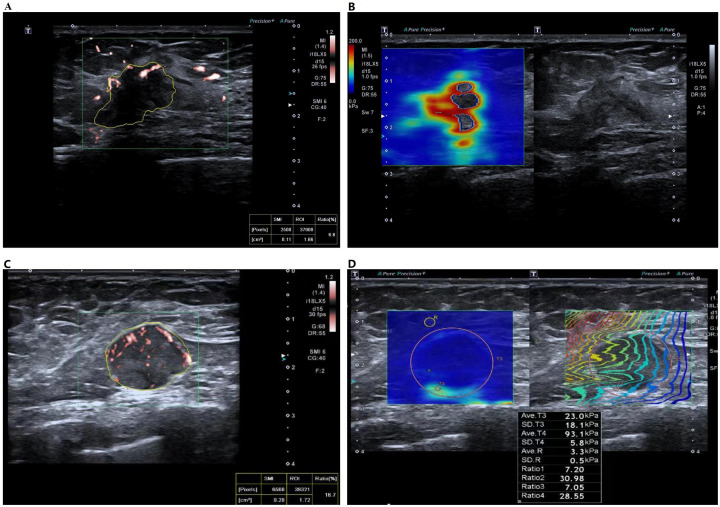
Multimodal ultrasound images of non-TNBC and TNBC cases. (A) Non-TNBC showing a vascular index (VI) of 6.6% and (B) the stiff rim sign on SWE. (C) TNBC showing a VI of 16.7% and (D) relatively homogeneous elasticity on SWE.
